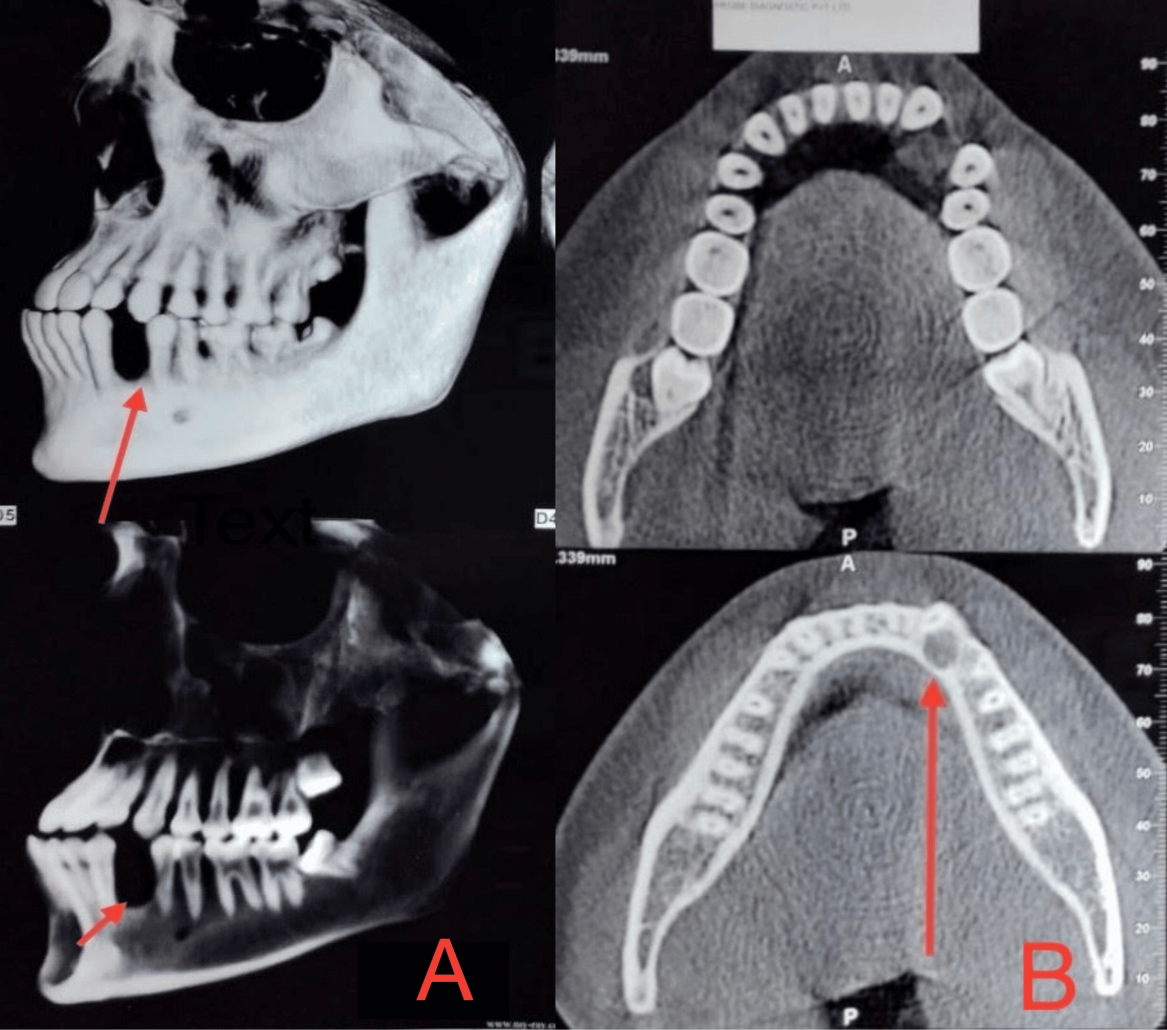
CBCT showing bone loss pattern (A and B) Saucerization or peripheral cupping of the dentoalveolar segment without affecting the basal bone. CBCT: cone-beam computed tomography
